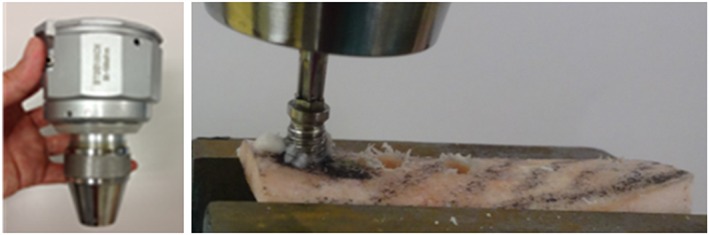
Digital torque gauge and regions of implantation. Ultrasound propagated the distal parts of the bone samples, and implants were inserted into the proximal, middle, and distal part of these parts
